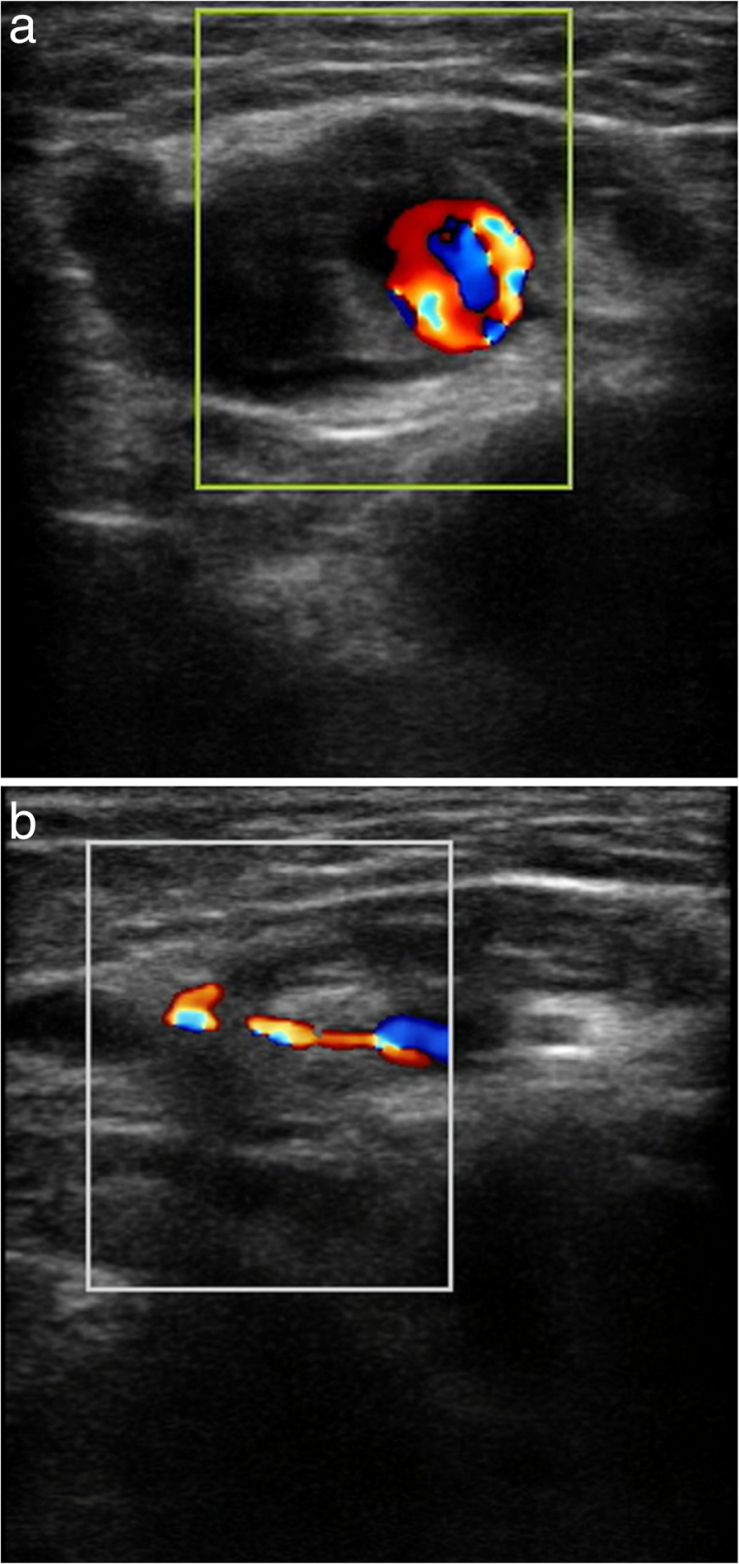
Doppler ultrasonography over the posteromedial aspect of the left knee. a A well-defined mass with bidirectional flow was shown. b The size of the mass decreased under compression with the ultrasound probe
